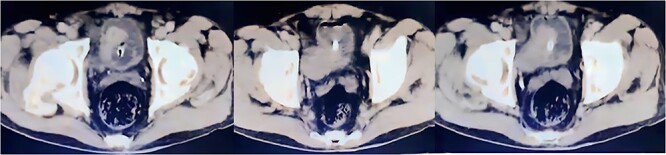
A CT scan of the pelvis reveals the location of bladder cancer.
